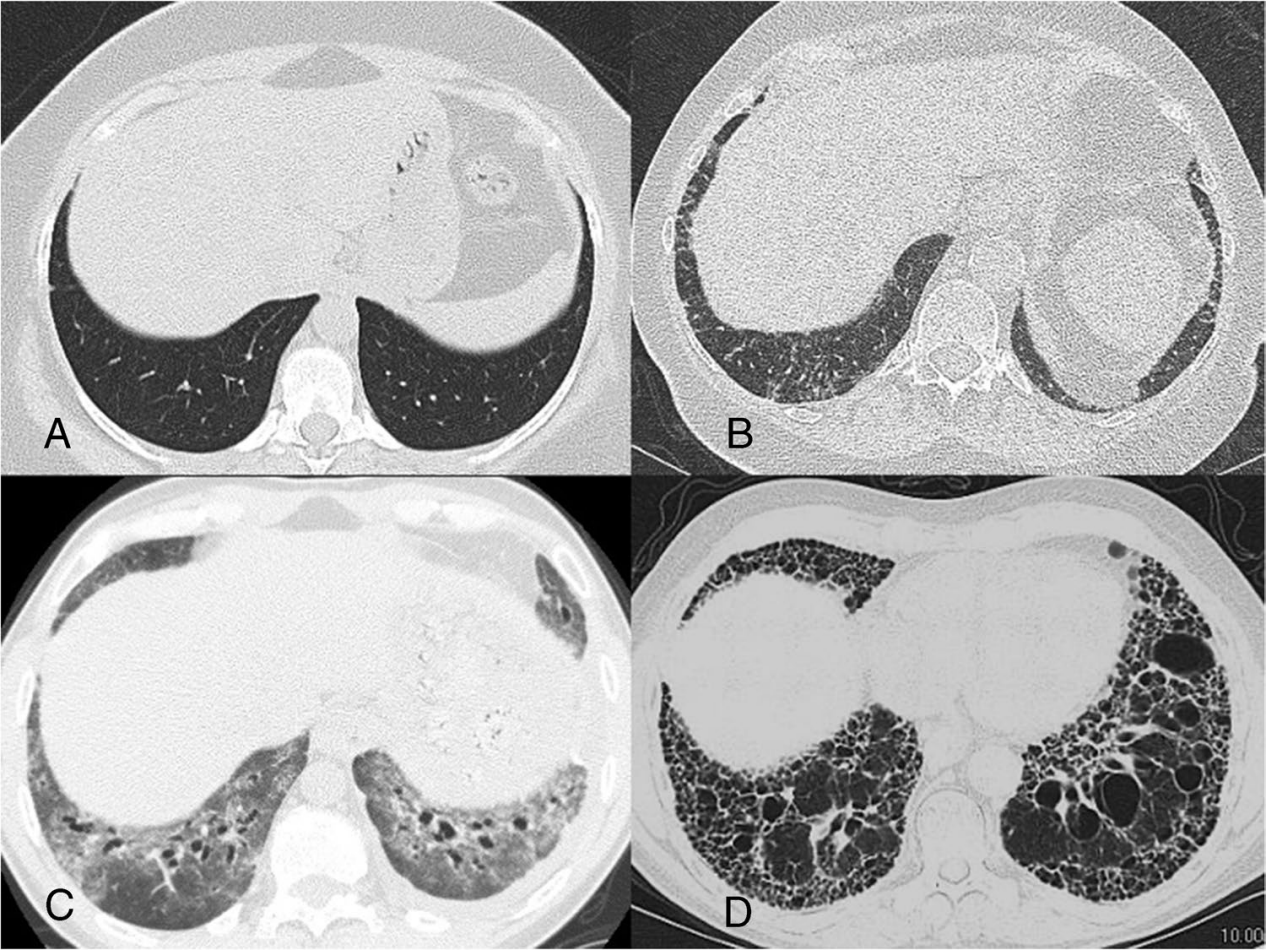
Examples of imaging patterns seen in patients with SSc. a Axial HRCT image from the normal lung bases of a 55-year-old woman undergoing staging for pancreatic cancer. The only visible structures are bronchovascular bundles and pleural reflections; there are no interstitial lung abnormalities. b Early fibrotic ILD in a 47-year-old woman with SSc. There are many crossing lines (reticulations), indicating early fibrotic lung disease. It is difficult to apply a specific pattern at this early stage. c An NSIP pattern in a 52-year-old woman with SSc. The pattern is characterized by ground-glass opacities, traction bronchiectasis, and subpleural sparing. d A UIP pattern of fibrotic ILD in a 59-year-old man with SSc. There are extensive reticulations and honeycombing throughout the basilar and peripheral lungs
